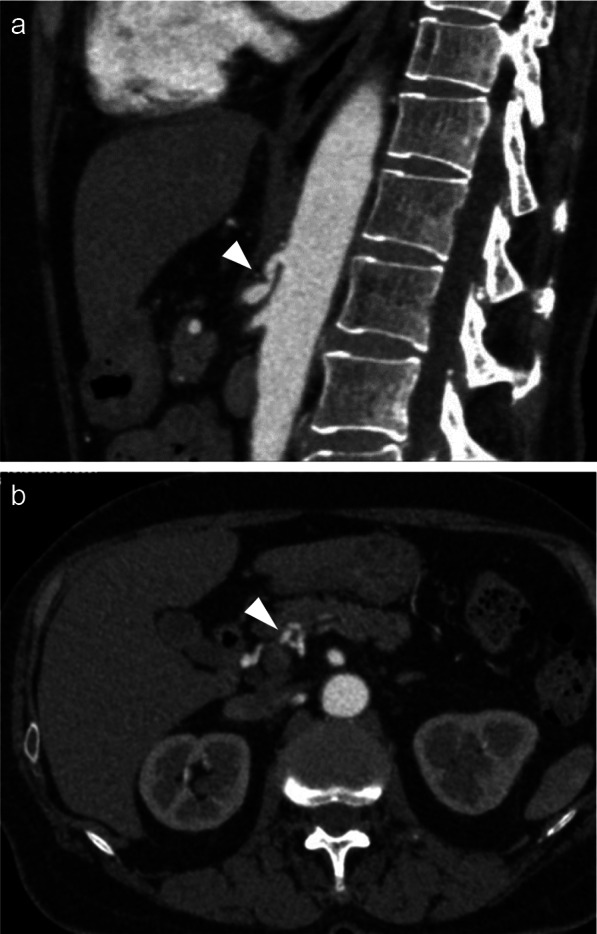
Preoperative abdominal contrast-enhanced computed tomography. a Sagittal image demonstrating celiac artery stenosis due to compression from the cranio-ventral side (arrowhead). b Axial image demonstrating collateral blood vessels around the pancreatic head (arrowhead)
